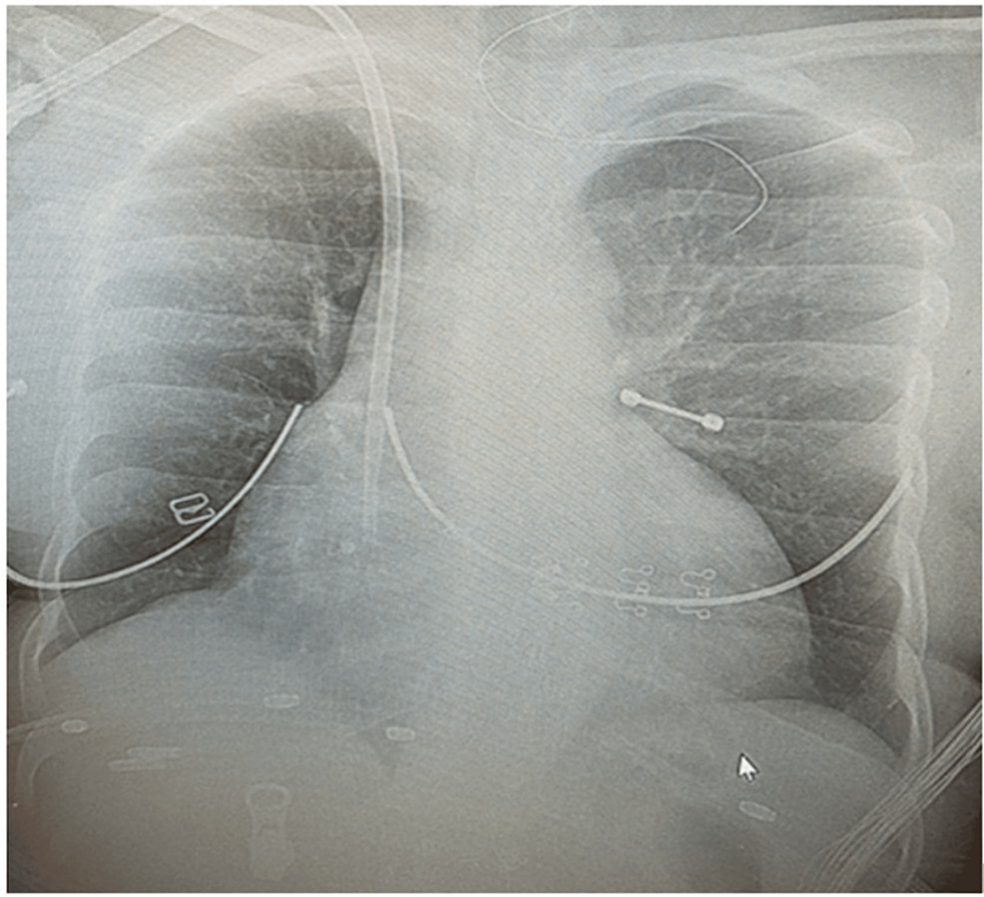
Chest X-ray in the current presentation (2023) showing cardiomegaly with dilation of the atrium. There is non-specific ground opacification with infectious or inflammatory etiology in the lungs.
